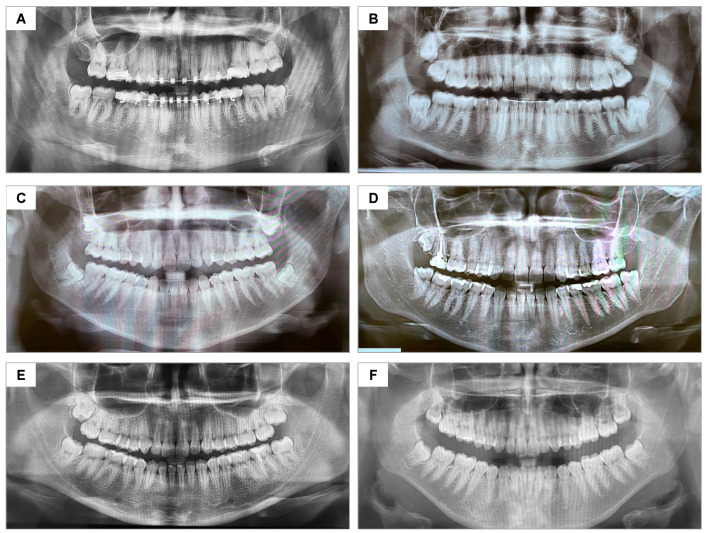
Panoramic radiographs representing the similarity in positioning and surgical difficulty of the lower third molars: (A) participant #18—A.G.S.J; (B) #7—M.P.M.B.; (C) #6—M.C.G.; (D) #4—J.A.A.L.; (E) #3—K.B.S.; (F) #11—B.V.R.
